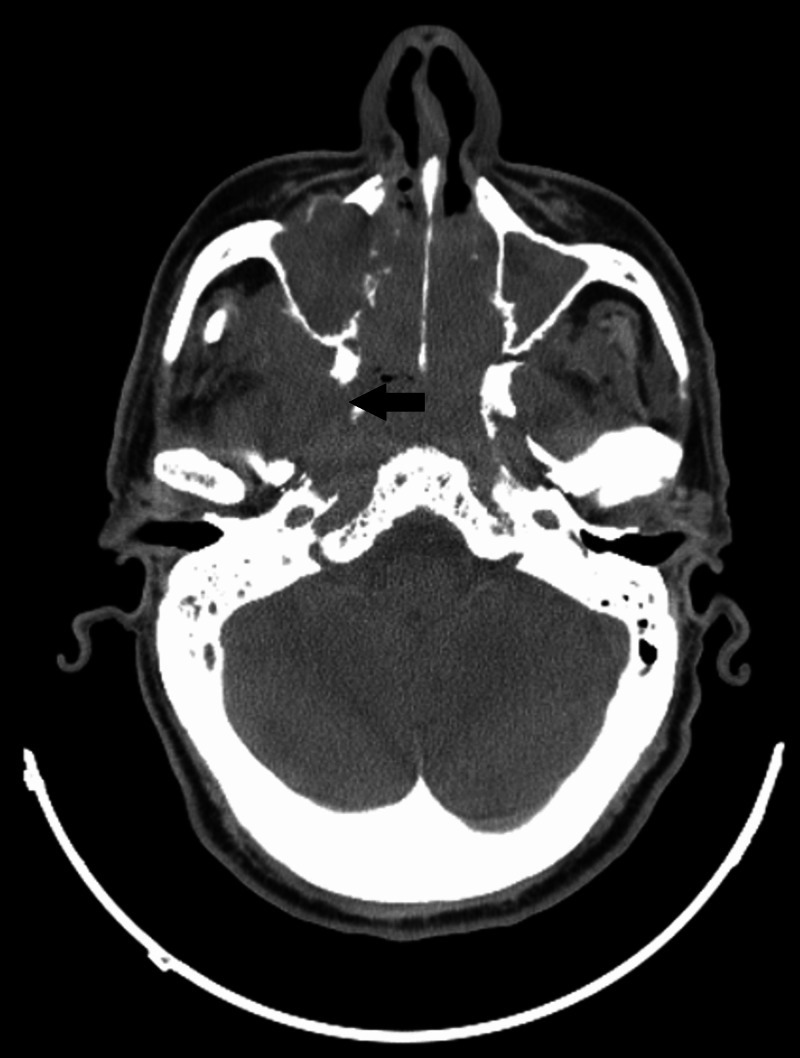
CT scan depicting the tumor (black arrow) from the height of the ethmoidal air cells to the ground of the oropharynx eroding different bones of the area.
